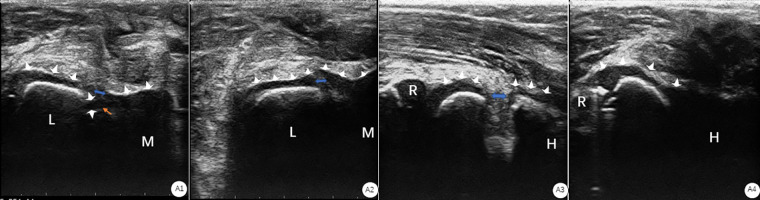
(A1) Ultrasonography on the transverse plane showed that the cartilage hinge (white arrow) was broken (blue arrow), the “step sign” was seen at the broken end, and the triangular bone fragment (yellow arrow) at the broken end was free from the cartilage articular surface. (Note: L the humerus Lateral condyle; M: the medial condyle of the humerus). (A2) Ultrasonography on the transverse plane showed that the cartilage hinge (white arrow) was broken (blue arrow), and the “step sign” was seen at the broken end. (A3) Ultrasonography on the coronal plane showed that the cartilage hinge (white arrow) was broken (blue arrow), and the “step sign” was seen at the broken end. (Note: R: radial head; H: humeral shaft). (A4) Ultrasonography on the coronal plane shows the continuity of the cartilage hinge (white arrow). (Note: it radial head; humeral shaft).
